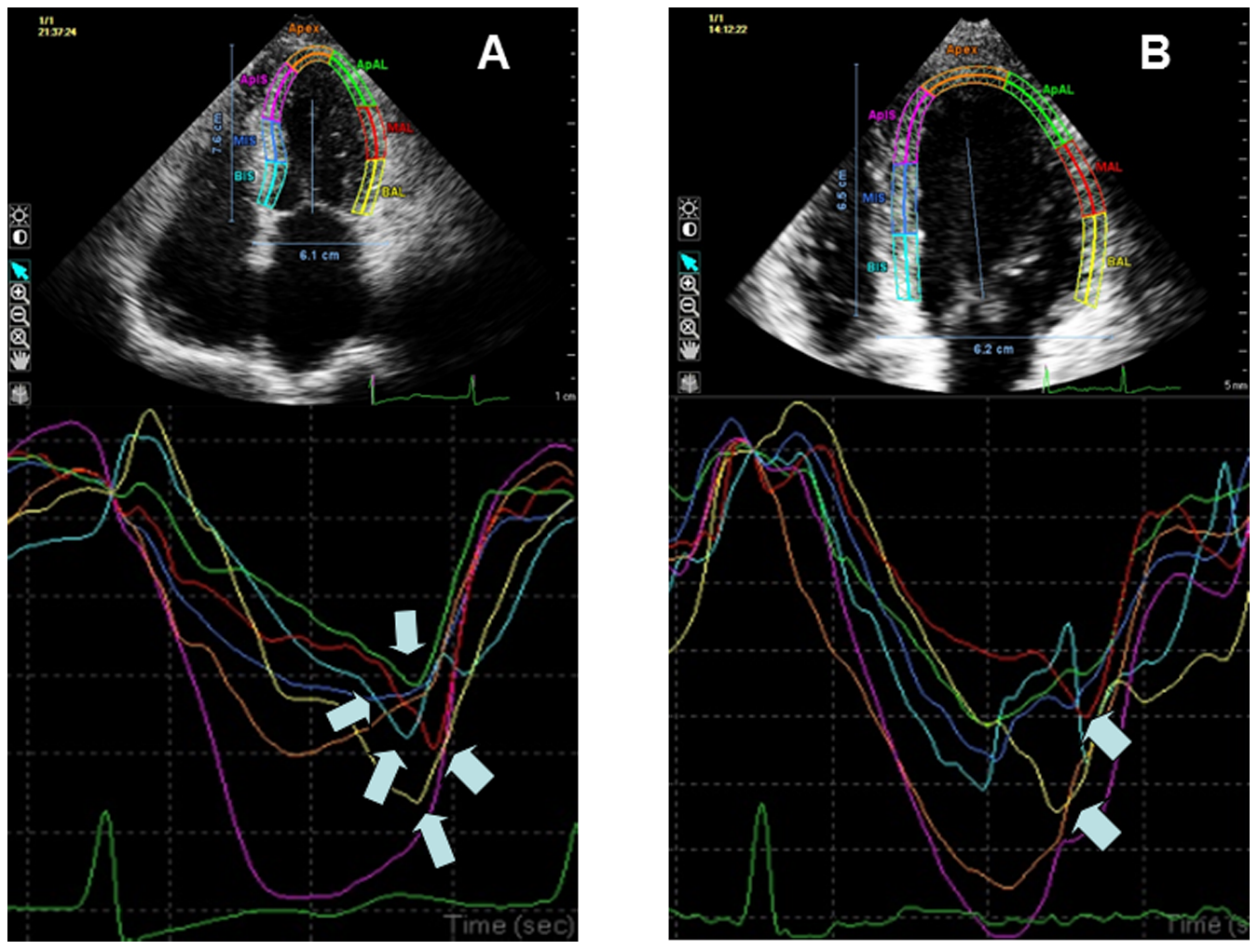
Strain curves from the apical 4-chamber view at baseline (A) and half a year after successful septal ablation (B) in a HOCM patient. The number of segments having PSS was significantly reduced at half a year after PTSMA. *Arrows*, Strain curves showing postsystolic shortening (PSS).
